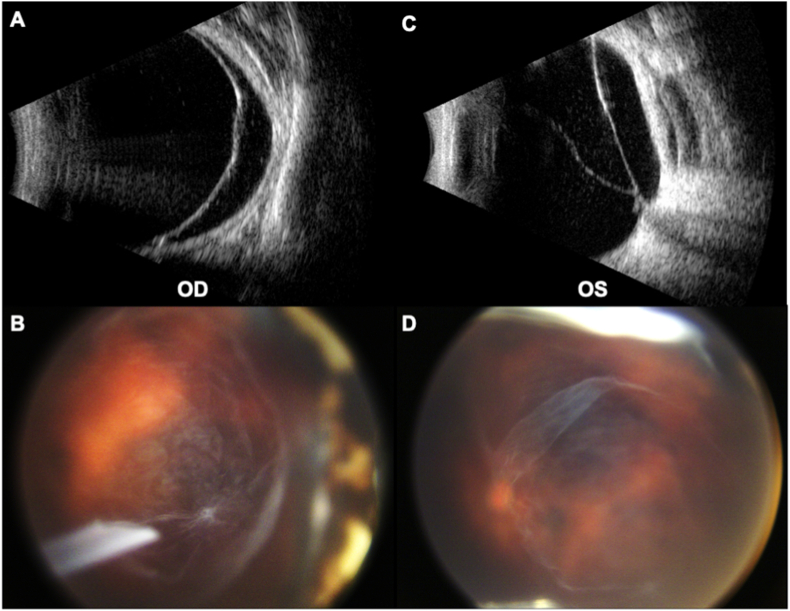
B-scan ultrasonography of demonstrating retinal detachment in the right eye (A) and left (C) eyes at the time of their diagnosis. Fundus photography demonstrating vitreous veils in the right (B) and left (D) eyes.
